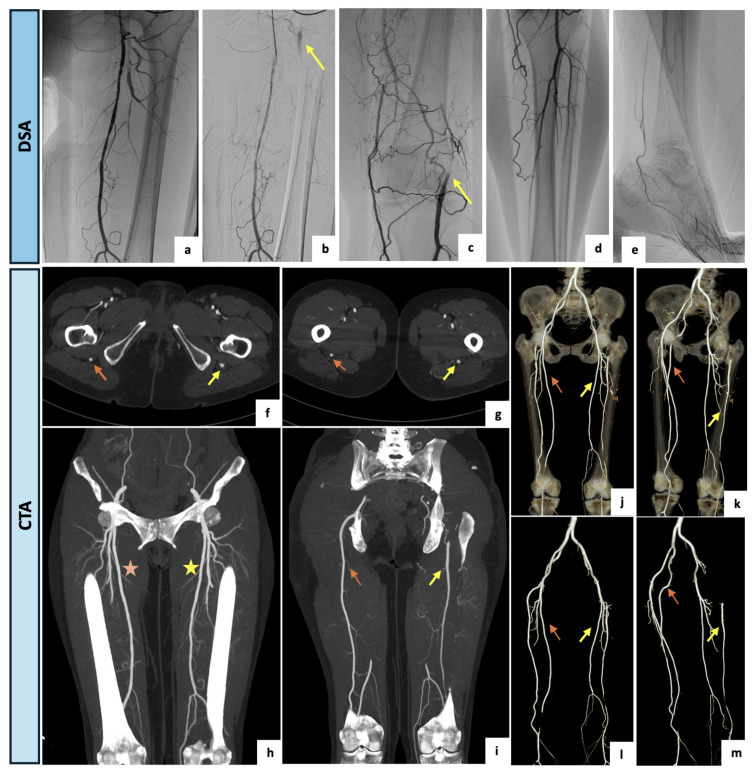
(a–e) Intraprocedural selective angiographic images obtained after a left antegrade common femoral access. (a–e) The angiograms demonstrate a diffusely hypoplasic and interrupted left SFA, lacking the usual continuation, and a popliteal artery supplied by collateral pathways originating from the distal small branches of the SFA and the deep femoral axis. (b,c) Surprisingly, an arterial vessel suggestive of incomplete PSA was retrograde perfused via deep femoral collateral pathways and visualized both proximally and distally as brief tracts (yellow arrows). (f–i) Axial and coronal maximal intensity projection views of the CTA confirming a bilateral PSA. On the left side, a type 2A PSA (yellow arrows) and a distal significant stenosis of the SFA (yellow asterisk) were detected. The right lower limb presented a type 1 complete PSA (orange arrow) along with a regular SFA (orange asterisk). (j–m) Three-dimensional reconstructions with and without bone structures. On the left side, a type 2a PSA with a proximal focal occlusion and re-habitation via gluteal collateral pathways, and a distal femoral significant stenosis was detected (yellow arrows). The right lower limb presented a type 1 complete PSA (orange arrows).
